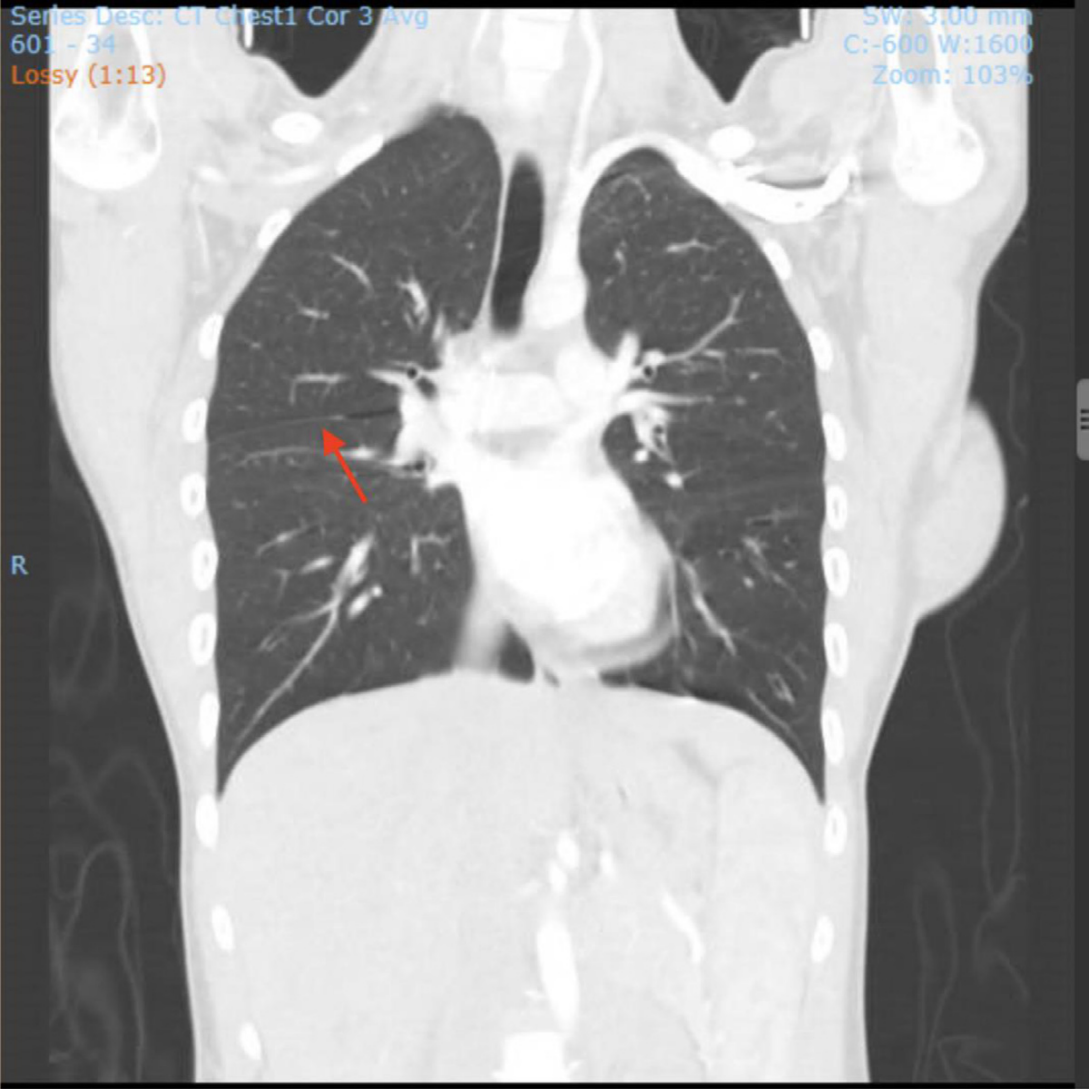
CT angiogram chest AP view showing the hyperlucency of primary spontaneous interlobar pneumothorax (red arrow).
